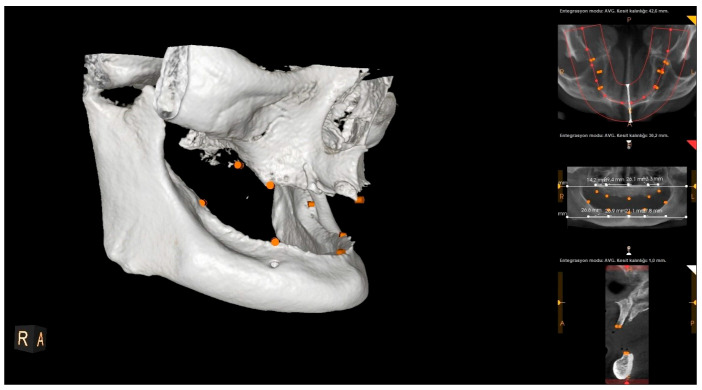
Landmarks on a CBCT image (right side view).
